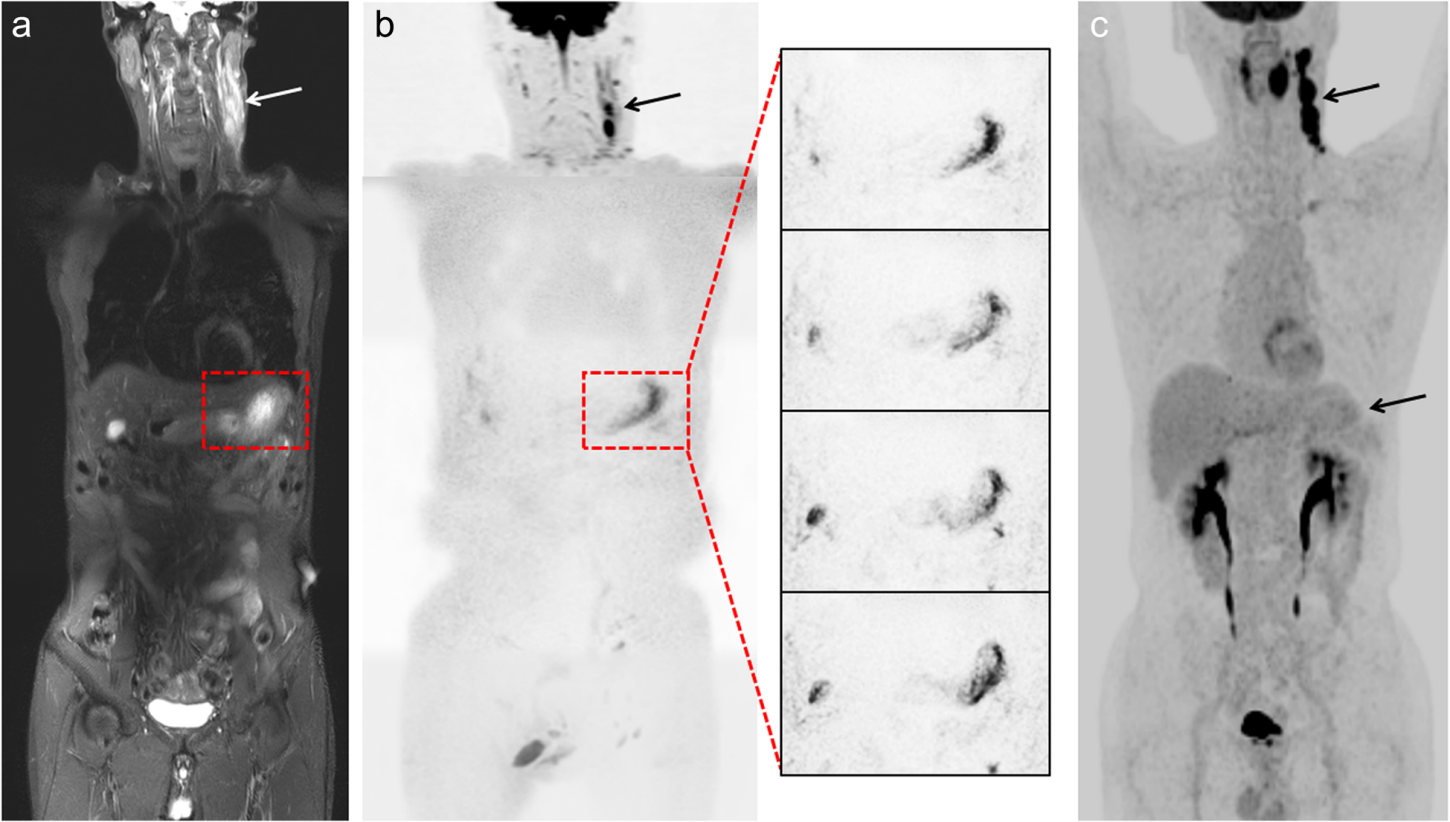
A 59-year-old man with MALToma of pretherapeutic stage IV. a Coronal whole-body T2-STIR and b DWIBS show lymphoma involvement of the stomach (dashed square) and lymph nodes in the neck (arrow). Magnified DWIBS images show high signal intensity along the greater curvature of the gastric body. c Coronal whole-body ^18^F-FDG-PET/CT MIP do not show 18F-FDG uptake in the corresponding area of stomach. DWIBS = diffusion-weighted imaging with background body signal suppression, T2-STIR = T2-weighted short-tau inversion recovery, ^18^F-FDG-PET/CT = 18F-fluorodeoxyglucose-positron emission tomography-computed tomography, MIP = maximum intensity projection
